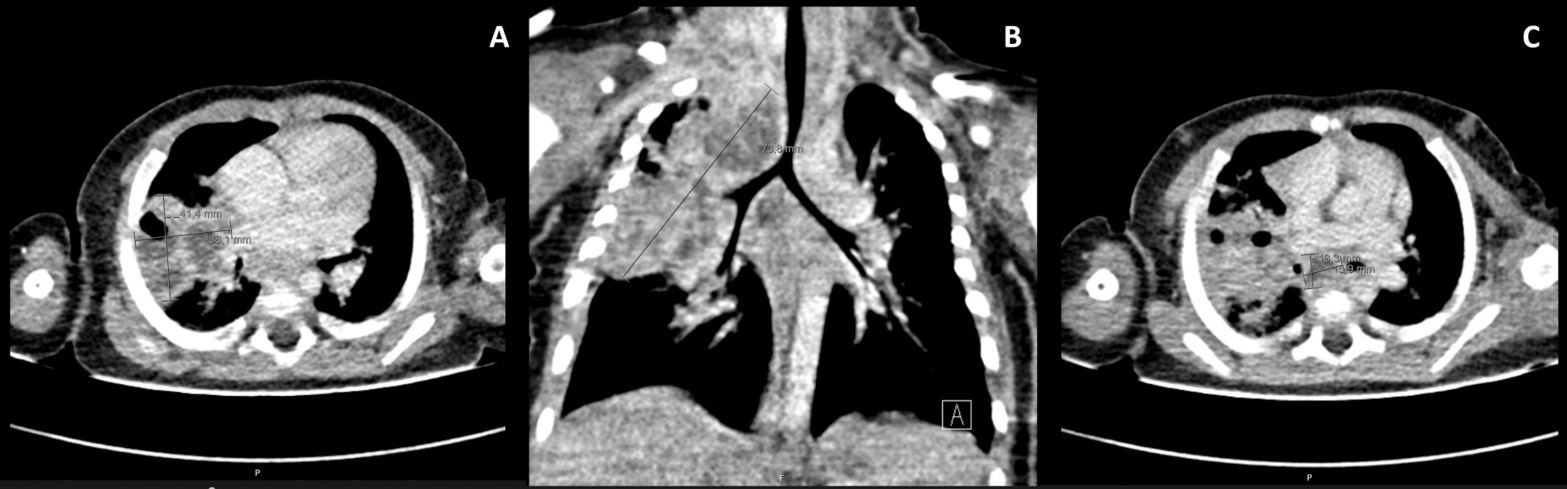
Contrast-enhanced thoracic computed tomography Contrast-enhanced thoracic computed tomography scan revealing in the upper right lobe a voluminous consolidation measuring 74x41x38mm (A, B) with areas of cavitation and contiguous with necrotic lymph nodes in the right hilar and mediastinal regions, the largest located in the subcarinal area measuring 16x13mm (C).
